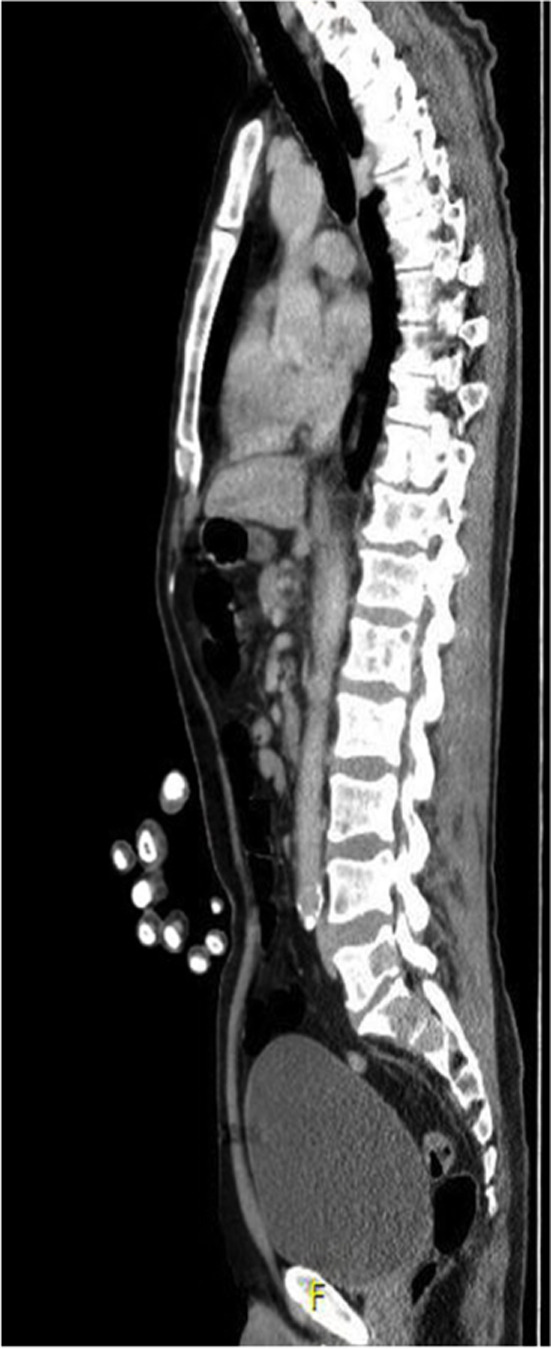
Sagittal section of CT Scan post‐CSF analysis, performed due to suspicion of neoplastic meningitis. Multiple vertebral metastases demonstrated
